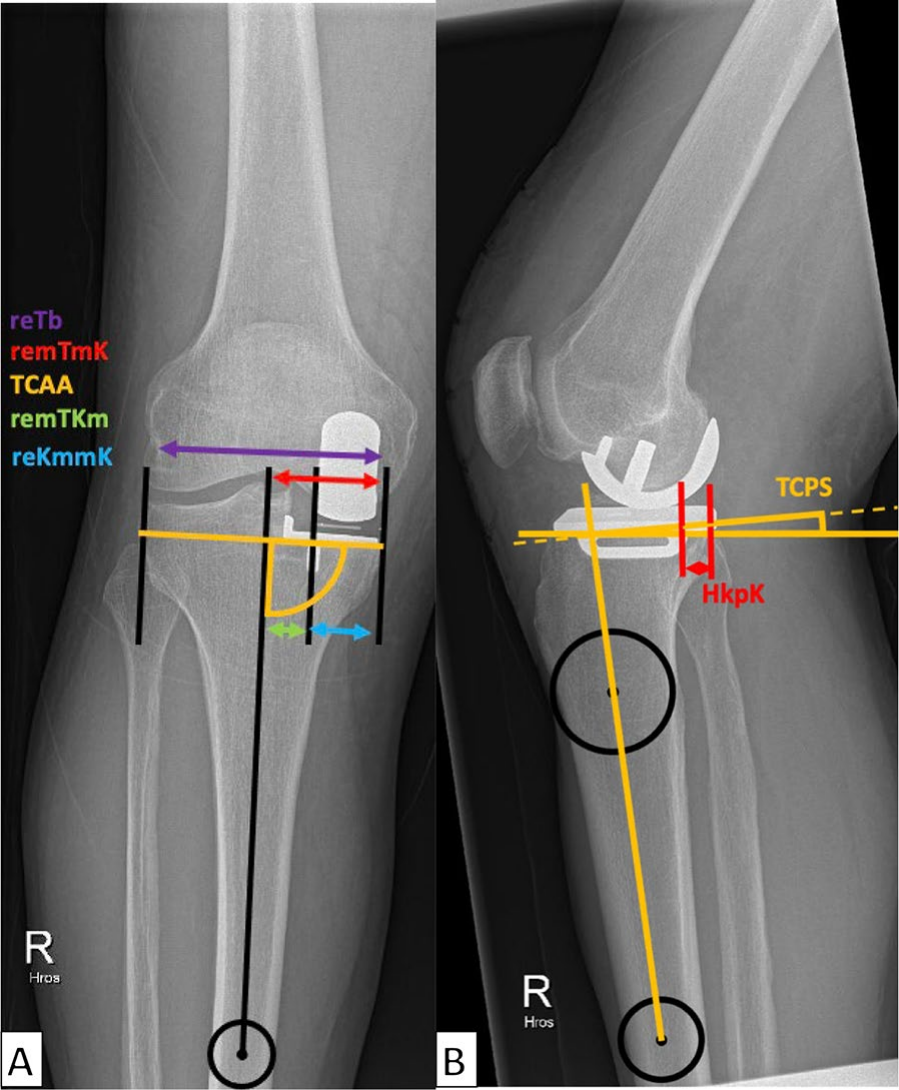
Postoperative measurements. X-ray was taken in a weight-bearing situation: width of the tibiaplateau (reTb, violet), medial tibiaplateau (remTmK, red), tibial component alignment angle (TCAA, yellow), distance from mechanical tibial axis to implant keel (remTKm, green), and distance from keel to medial tibial cortex (reKmmK, blue) were measured (A); tibial component sagittal posterior slope (TCPS, yellow) and distance from keel to posterior cortex (HkpK, red) at resection level were obtained (B)
